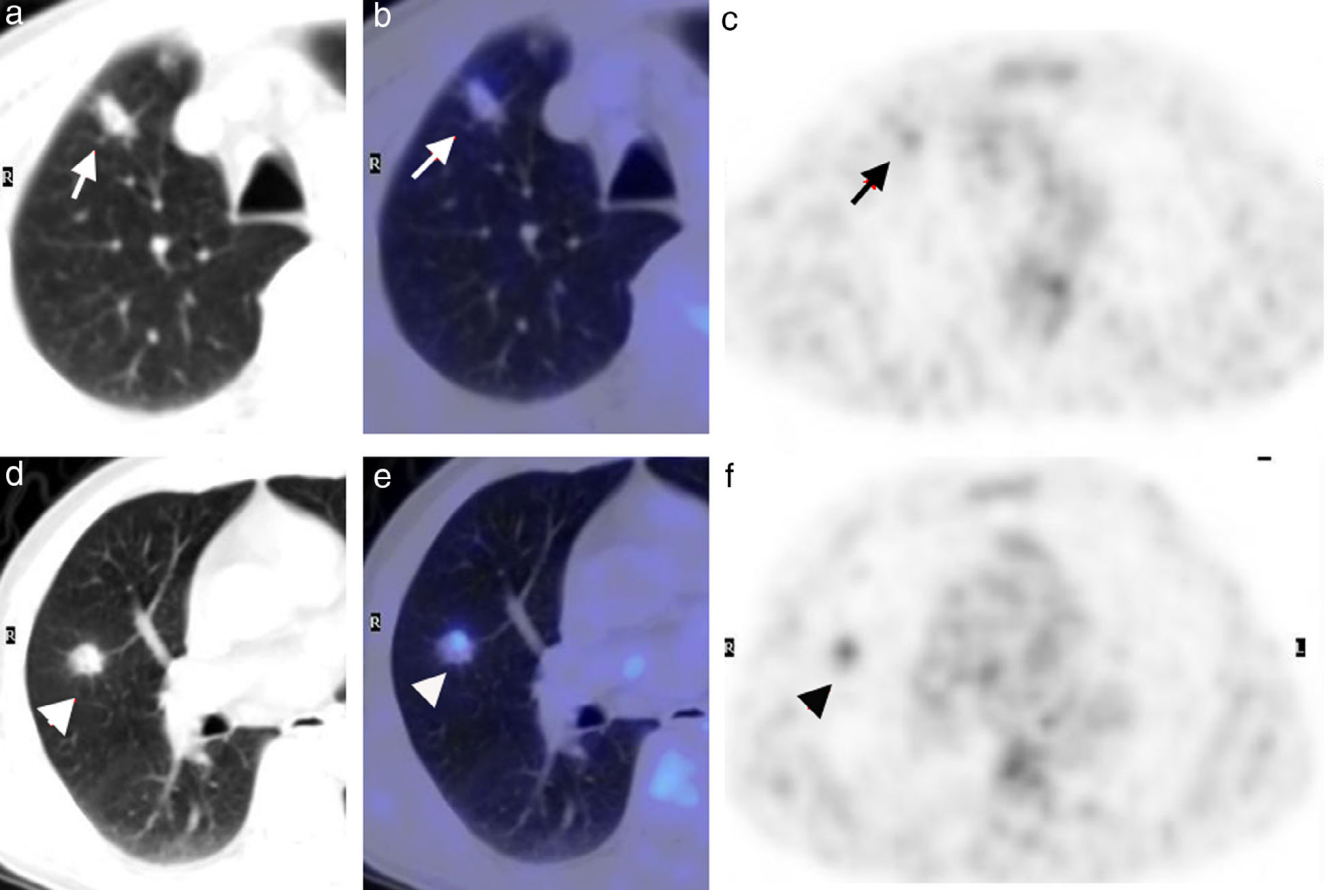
Two atypical PSPs were found in the right upper lobe (a, b) and right middle lobe (d, e) in one patient (Case 3 in Table 1). The two lesions showed moderate and intense uptake of FDG, respectively (c, f).
